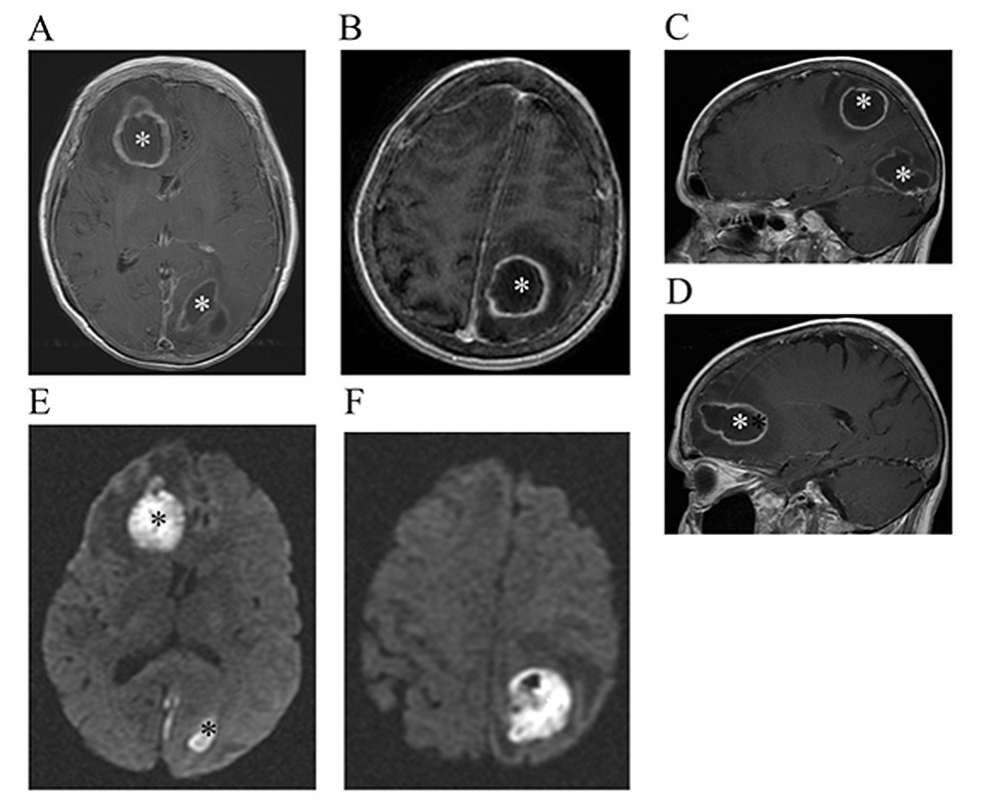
Initial MRI with contrast. Initial MRI of the brain with contrast (A-D) and DWI (E-F) showing the three large enhancing lesions in frontal, occipital, and parietal areas with DWI signals consistent with the presence of brain metastasis or abscesses (*). MRI: magnetic resonance imaging; DWI: diffusion-weighted imaging
